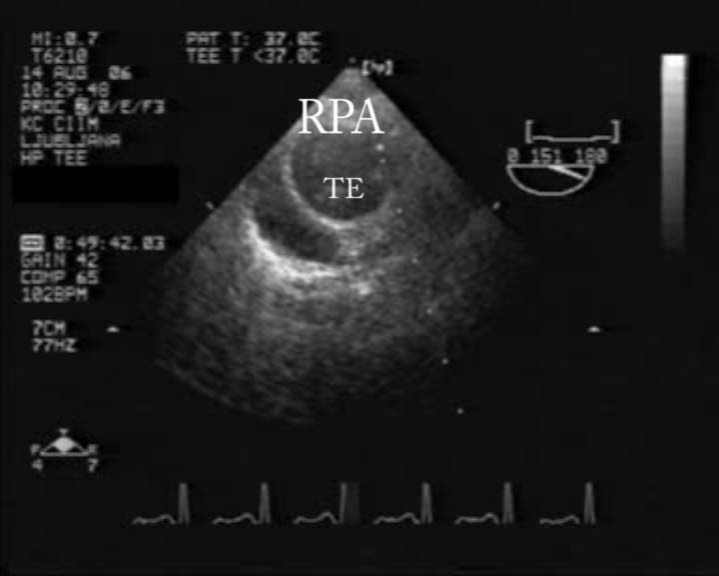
Transesophageal echocardiography showing trombemboli in right pulmonary artery. Tromboemboli (TE) in right pulmonary artery (RPA)
